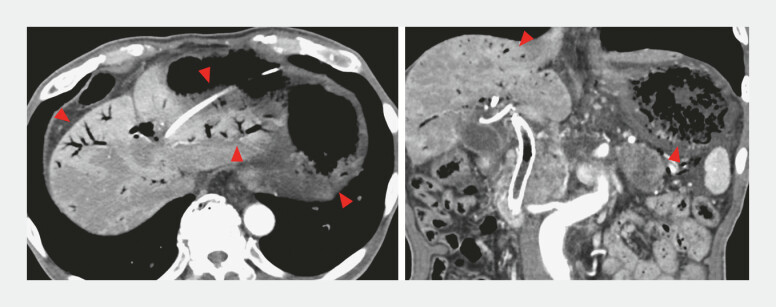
Contrast-enhanced computed tomography (arterial phase) at emergency readmission revealed extensive gas accumulation within the gastric wall and portal venous system (red arrowheads). Vital signs were stable, and there were no peritoneal irritation signs. Laboratory tests showed a normal white blood cell count, a mildly elevated C-reactive protein level of 3.01 mg/dL, and total bilirubin of 2.8 mg/dL. Acute cholangitis was ruled out based on imaging and laboratory results, and biliary drainage was considered adequate.
